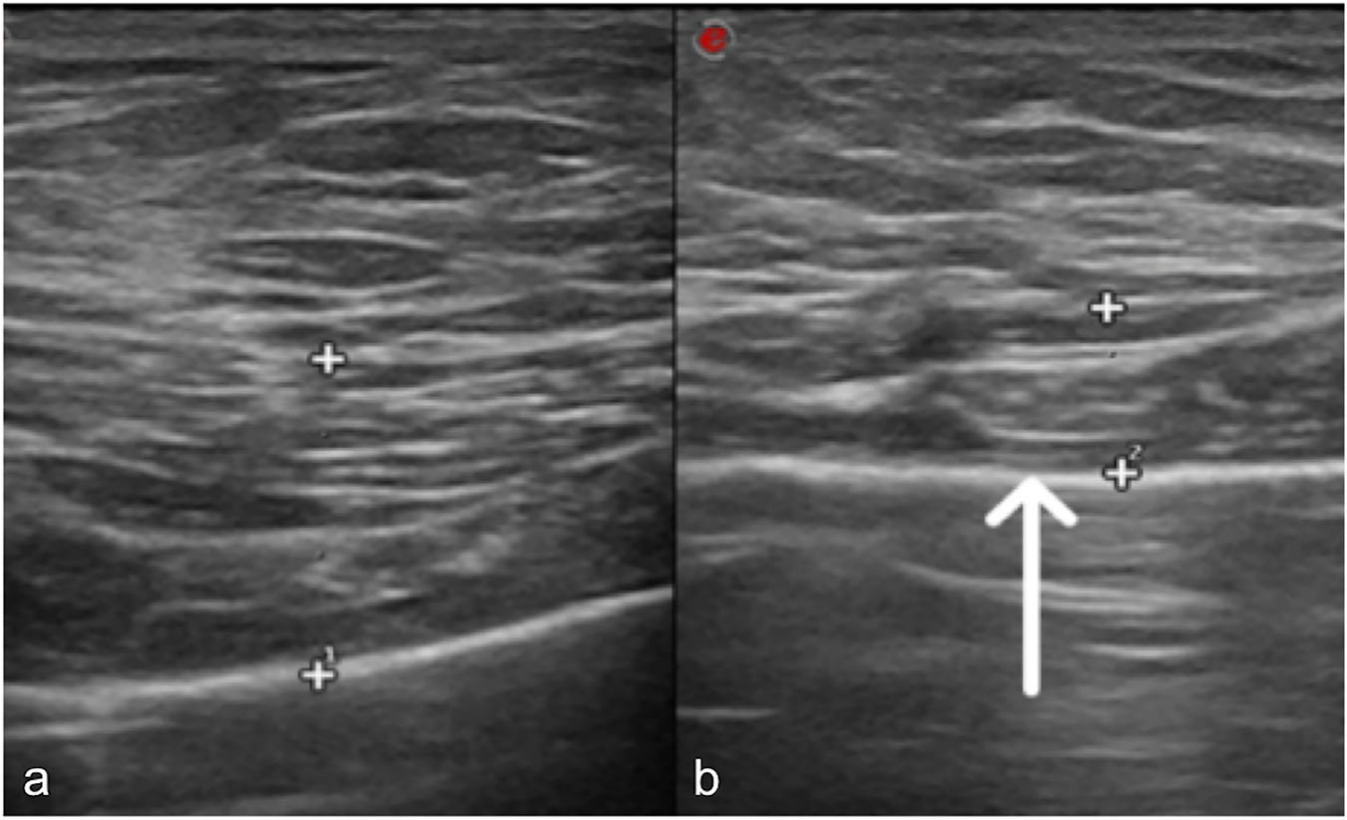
A 12-year-old male affected by Poland syndrome. Ultrasound images reveal normal pectoral muscle on the right side (a) and left pectoral muscle atrophy (white arrow in b)
